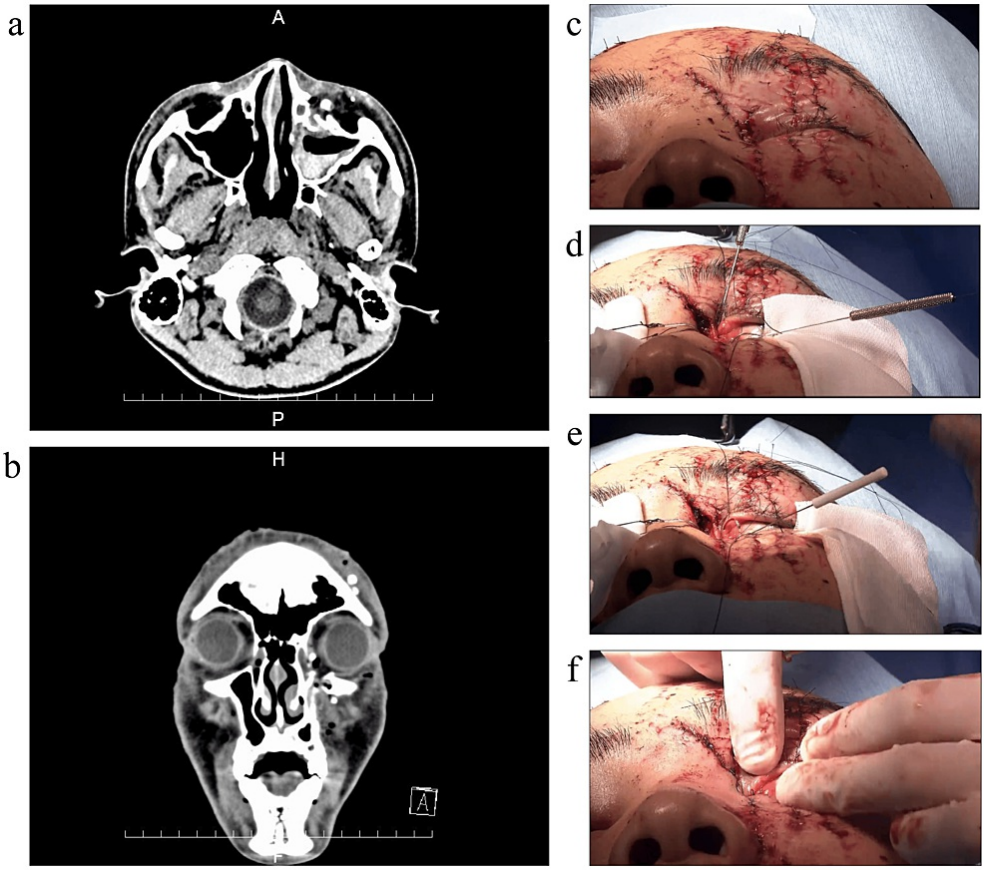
Case 1 (a, b) Preoperative CT images show depression of the anterior aspect of both maxillary sinuses and bilateral zygomatic fractures as well as high intensity areas that appear to be multiple glass fragments; (c–f) Photo of the face taken during the surgery; (c) Before the incision. The wound was sutured in the emergency room the previous day; (d) The sutured wound of the medial canthus is opened. A bougie is inserted through the upper and lower lacrimal canaliculi into the proximal cut surface to confirm the presence of a hard stop; (e, f) A bicanalicular stent is inserted from the upper and lower lacrimal puncta toward the nasolacrimal duct, followed by wound closure Written informed consent for the publication of facial photographs was obtained from the patient.
